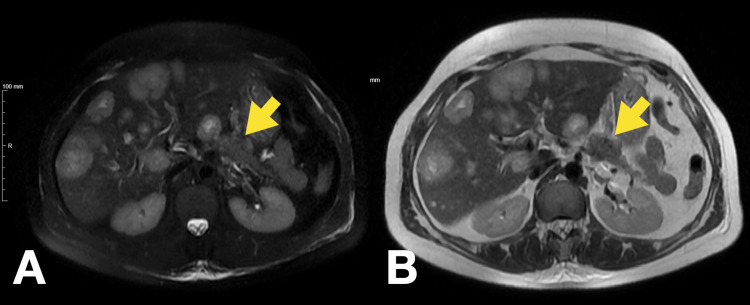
Abdominal MRI showing multiple liver nodules and solid nodular lesion of the pancreatic body T2 fat-suppressed (A) and T2-weighted MRI (B) images showing NET (yellow arrow) and liver metastases MRI: magnetic resonance imaging, NET: neuroendocrine tumor
